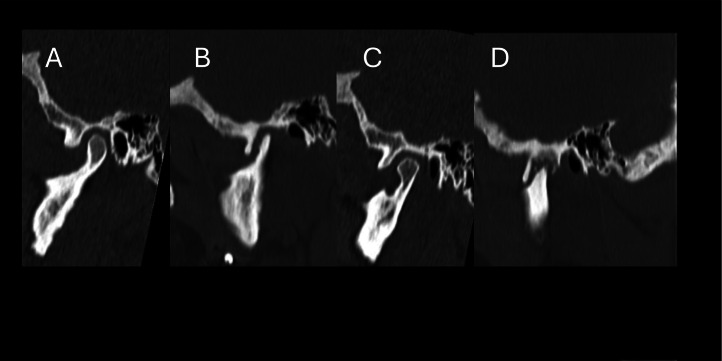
CT Image of TMJ. Sagittal view. (A) Right condyle before surgery. Normal condylar process (B) Right condyle 2 years after surgery. Incredible loss of bone volume in the heads of mandible and flattening of mandibular fossa (C) Left condyle before surgery. Normal condylar process and (D) Left condyle 2 years after surgery, incredible loss of bone volume in the heads of mandible and flattening of mandibular fossa.
